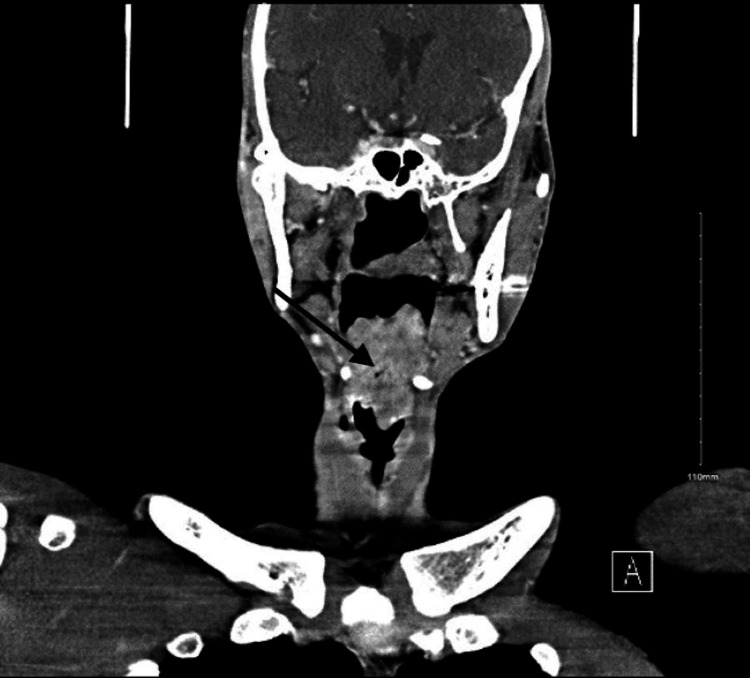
Contrast-enhanced computed tomography images (coronal view) showing a lobulated mass arising from the epiglottis and extending to the oro- and hypopharynx. The mass lesion causes near-complete obstruction of the upper airway.
